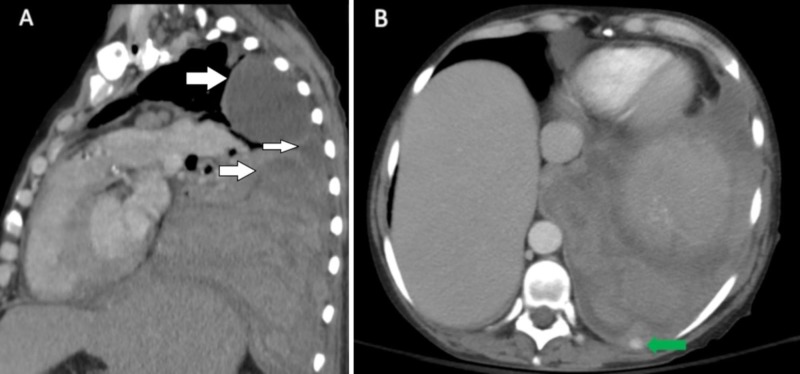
Multi-loculated pleural effusion and active bleeding on CT angiogram A) Coronal view from the computed tomography (CT) angiogram of the chest showing a multi-loculated pleural fluid collection in the left hemithorax (white arrows). B) Axial image from the CT angiogram showing extravasation of contrast (green arrow) compatible with active bleeding
